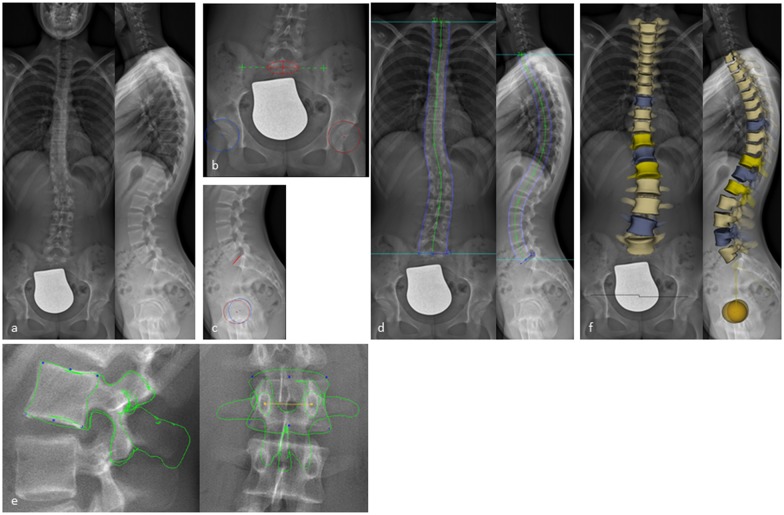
3D reconstruction from biplanar X-rays using sterEOS. (a) Biplanar X-rays performed with EOS in a standing position. (b,c) Digitalization of primary anatomical landmarks on the pelvis defining the position of the sacrum (red circle with a cross in the a.p. and red line in the lateral view) and the iliosacral joints (green crosses) as well as on both acetabuli (red and blue circles with a cross in the middle). (d) Shaping the spine from T1 to L5 using green control points. (e) Shaping every vertebral body (T1-L5) by identifying anatomical landmarks using blue and yellow control points on the vertebral bodies resulting in a 3D full spine model (f).
